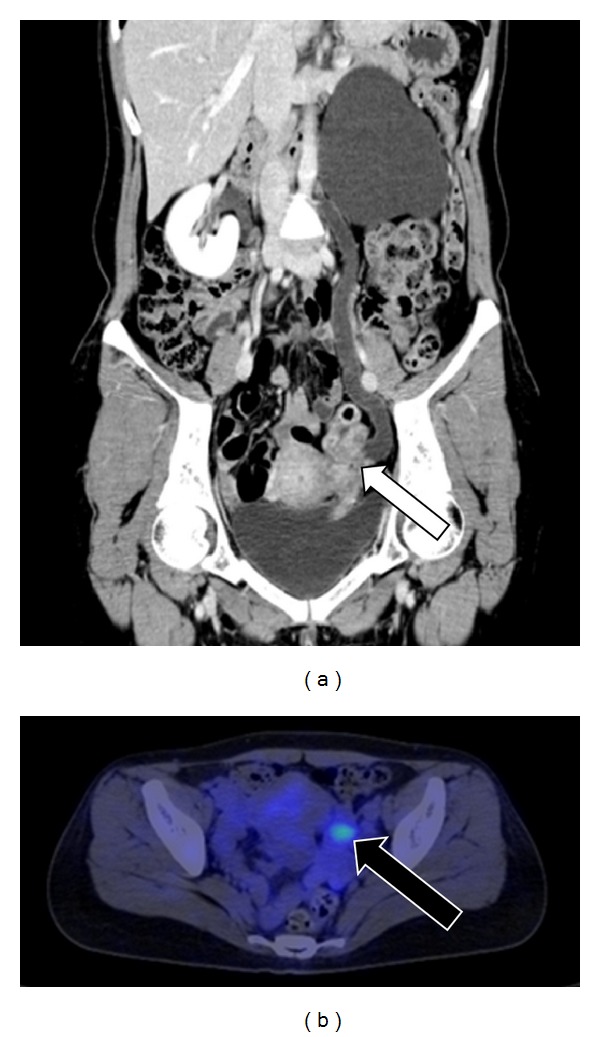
Preoperative image. (a) Coronal enhanced computed tomography (CT) showing an unclear mass that involved the left adnexa and ureter, indicated by an open arrow, along with severe left hydronephrosis and hydroureter. (b) ^18^F-fluorodeoxyglucose (^18^F-FDG) positron emission tomography/CT showing high ^18^F-FDG uptake at the internal iliac lymph node lesion, indicated by a closed arrow.
